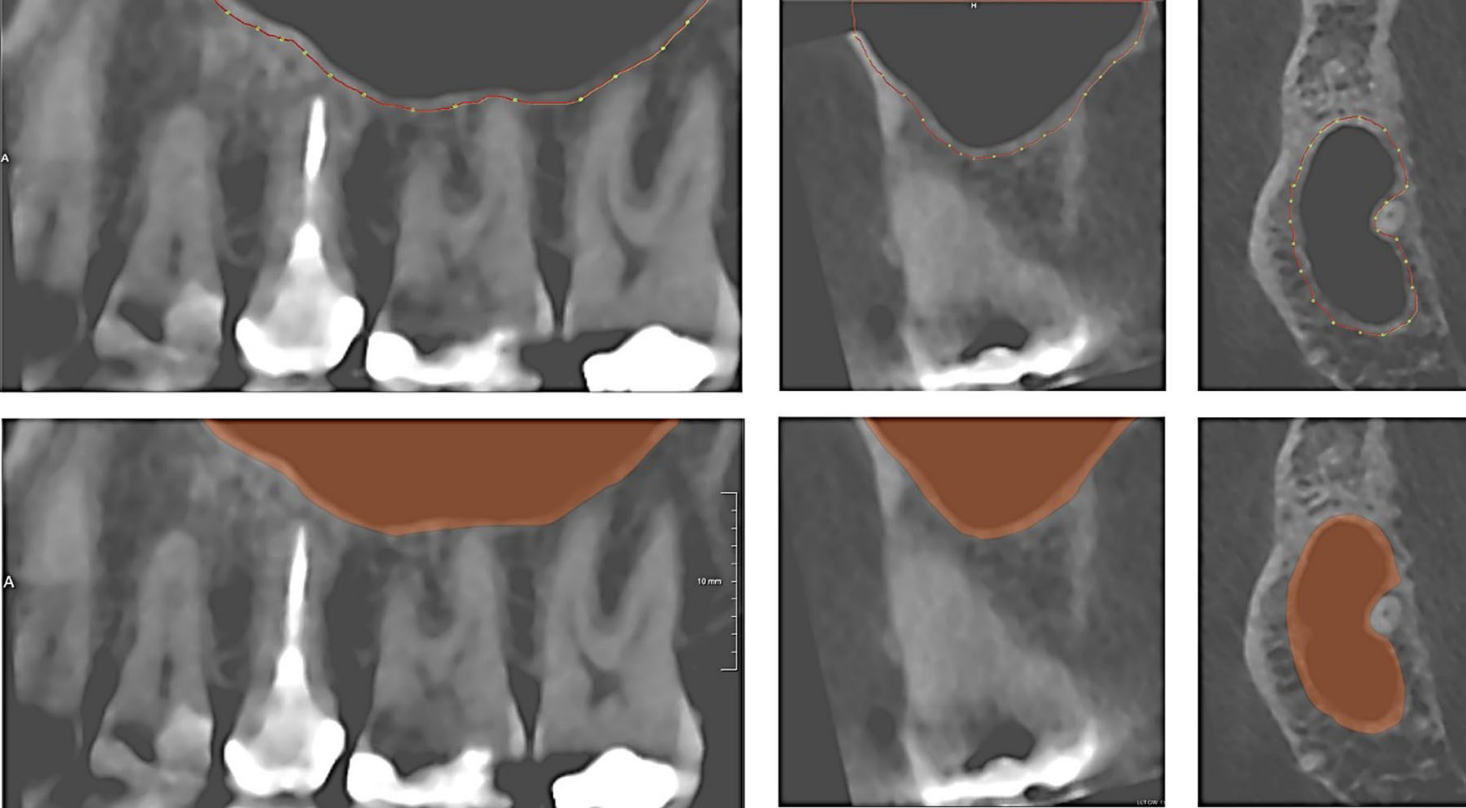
Segmentation of maxillary sinus and control of the suggested shape of the maxillary sinus in the three orthogonal planes (sagittal, coronal, and axial) in comparison to the shape of the maxillary sinus in the CBCT image. CBCT, cone‐beam computed tomography
